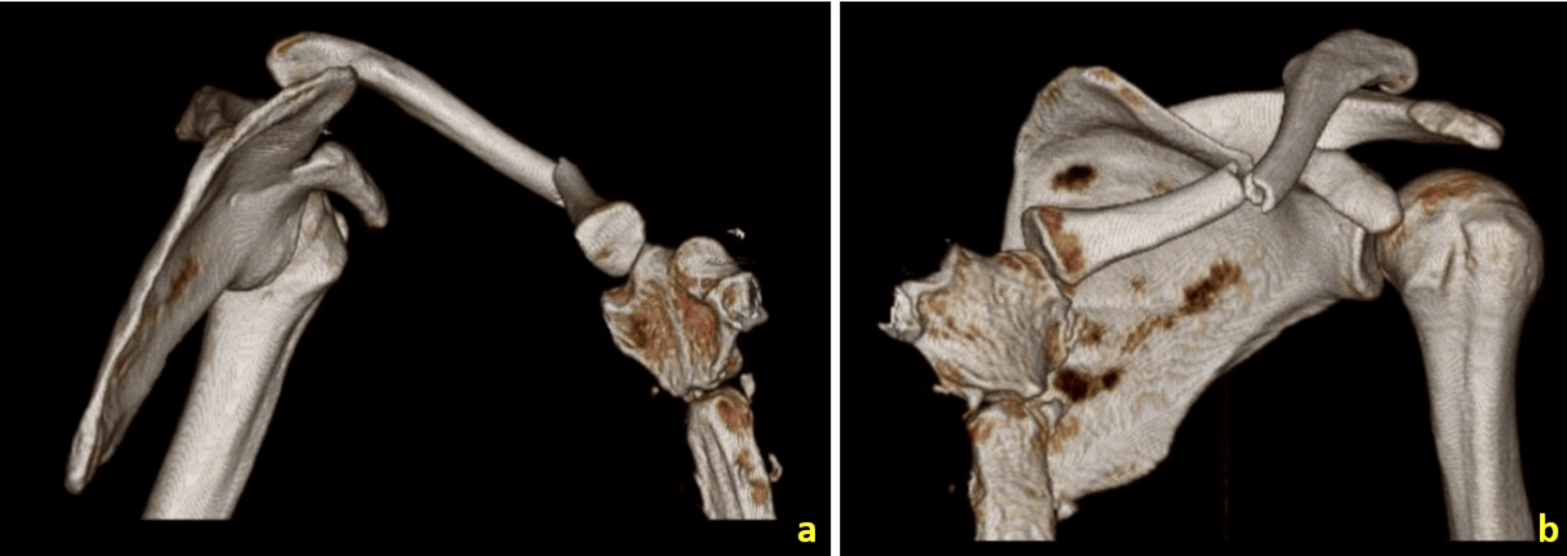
Computed tomography with a three-dimensional (3D) reconstruction of the left shoulder girdle. (a) Medial view showing posterior sternoclavicular subluxation. (b) Anteromedial view showing the displaced fracture with anterior angulation and high-grade acromioclavicular dislocation.
